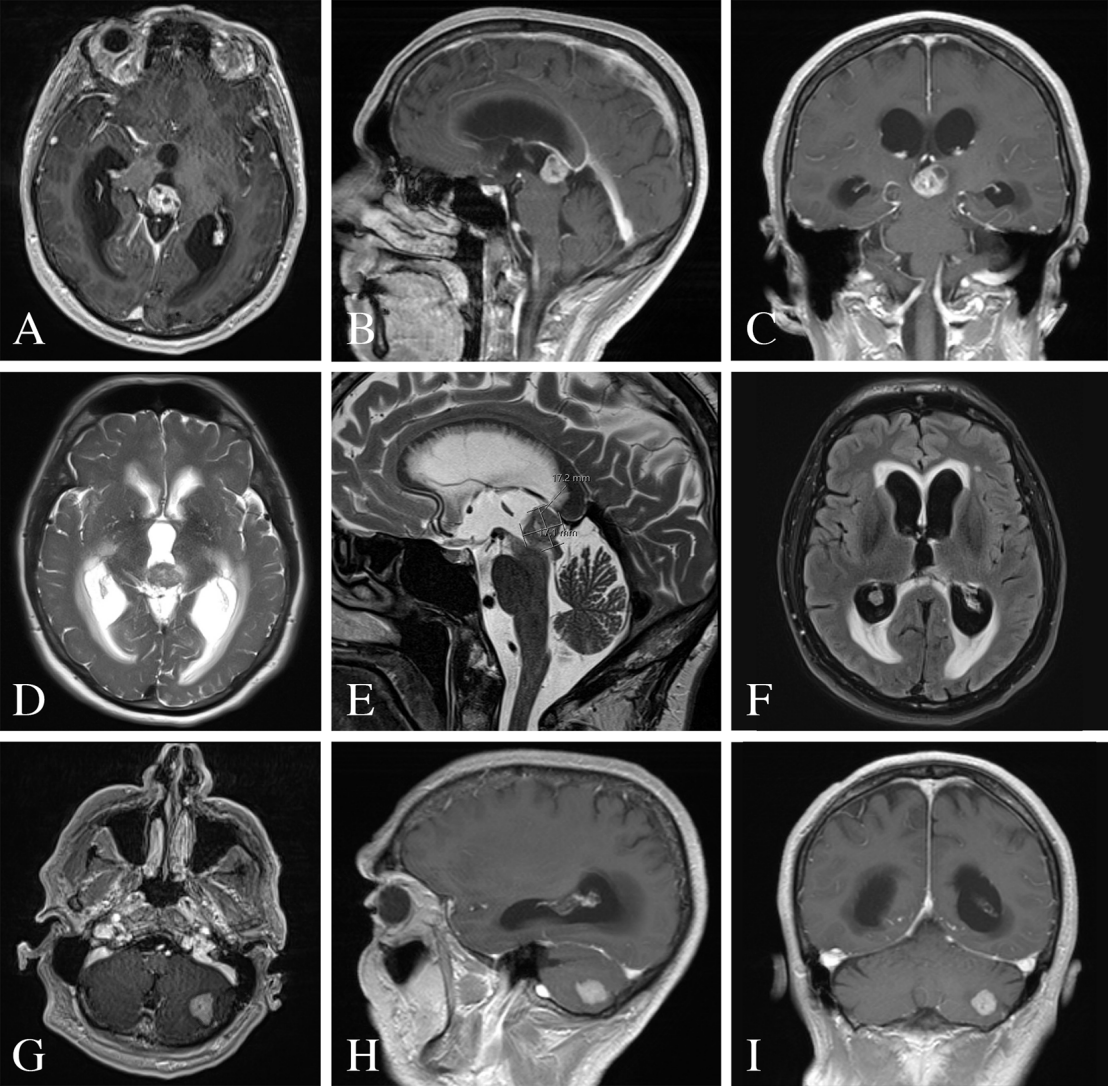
Pre-operative MRI of the brain. (A–C) MRI T1-weighted images with contrast demonstrating a heterogeneously enhancing lesion of the pineal gland. (D, E) MRI T2-weighted images with a T2 hypointense lesion with mass effect and compression of the cerebral aqueduct resulting in supratentorial ventricular dilatation and periventricular white matter signal abnormality. (F) MRI FLAIR image demonstrating periventricular transependymal flow of cerebrospinal fluid indicative of acute hydrocephalus. (G–I) MRI T1-weighted images with contrast demonstrating an enhancing lesion of the left cerebellar hemisphere.
